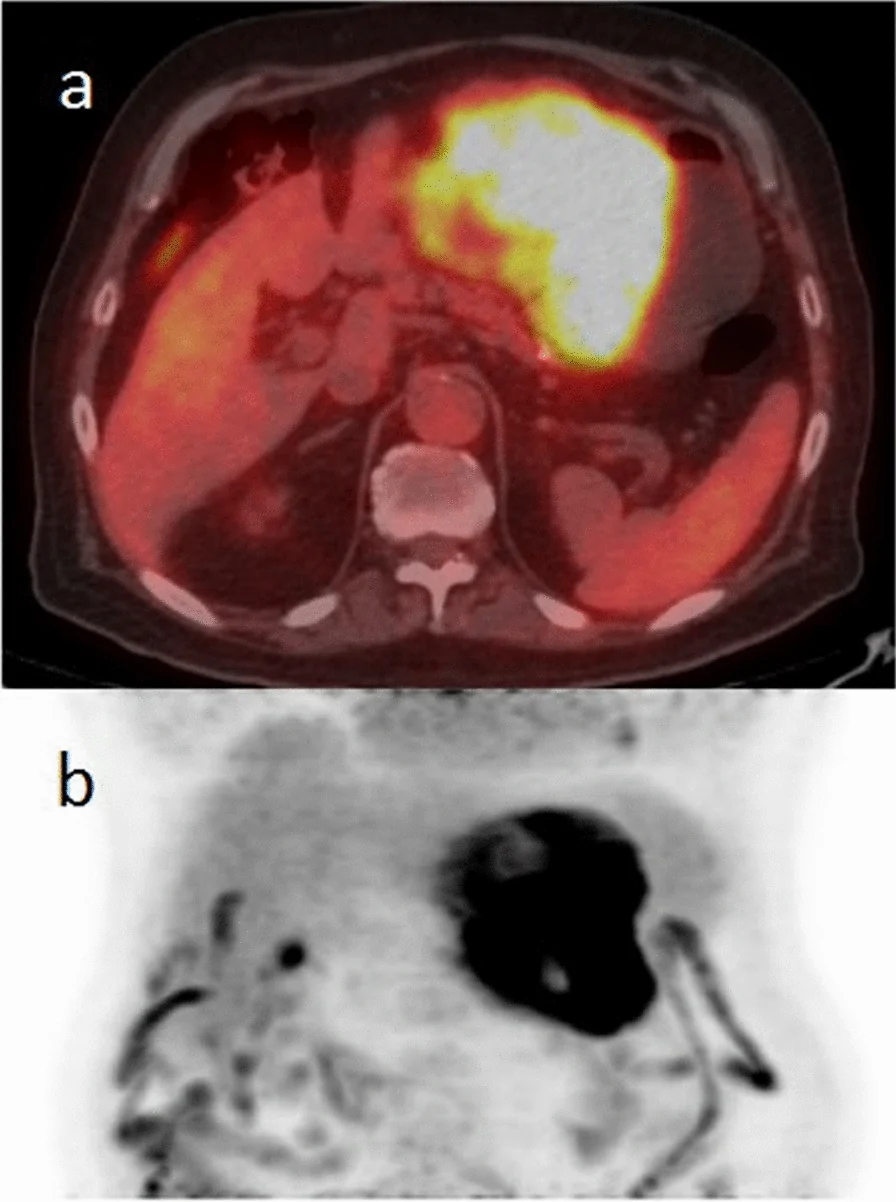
Positron emission tomography–computed tomography images of the gastric lesion. a Axial positron emission tomography–computed tomography demonstrating a hypermetabolic mass along the lesser curvature of the stomach. b Maximum intensity projection in 3D view revealing an irregular, FDG-avid mass in the same location
